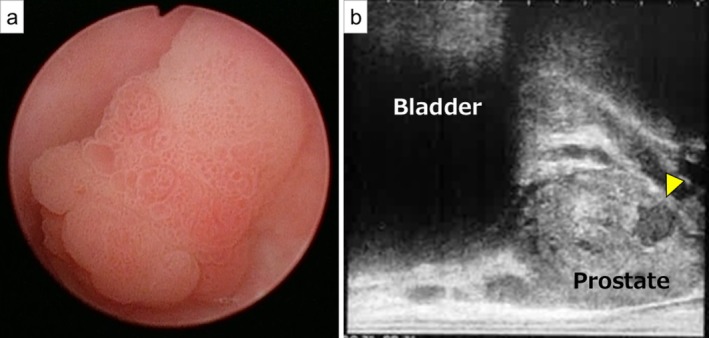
Intraoperative findings. (a) Cystoscopy showing a papillary tumor on the left lateral bladder wall. (b) Transrectal ultrasonography showing a hypoechoic mass within the prostate (arrowhead).
